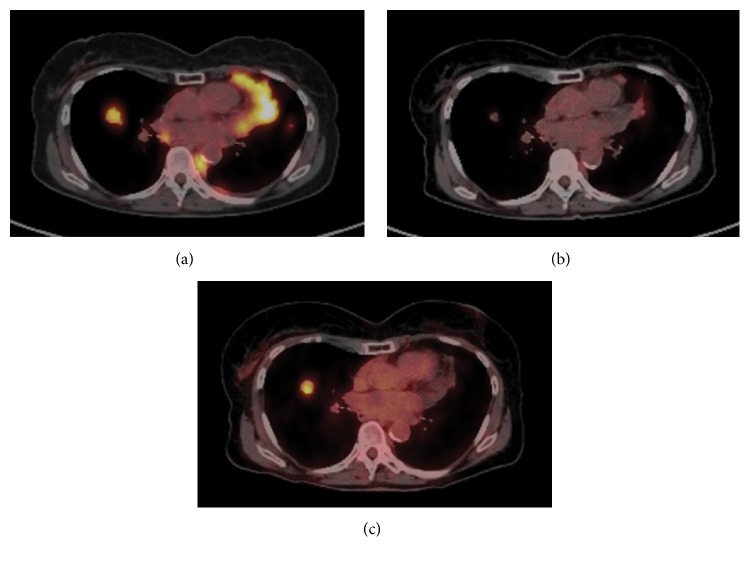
Representative example of a patient with oligoprogressive metastatic breast cancer. (a) PET scan of the central chest at the time of diagnosis of metastatic disease. (b) PET of the same area after 8 months of fulvestrant demonstrating resolution of the FDG avid disease. (c) Surveillance PET scan 6 months later demonstrating solitary site of progressive disease in the right lung. No other evidence of progressive disease was noted in this patient.
